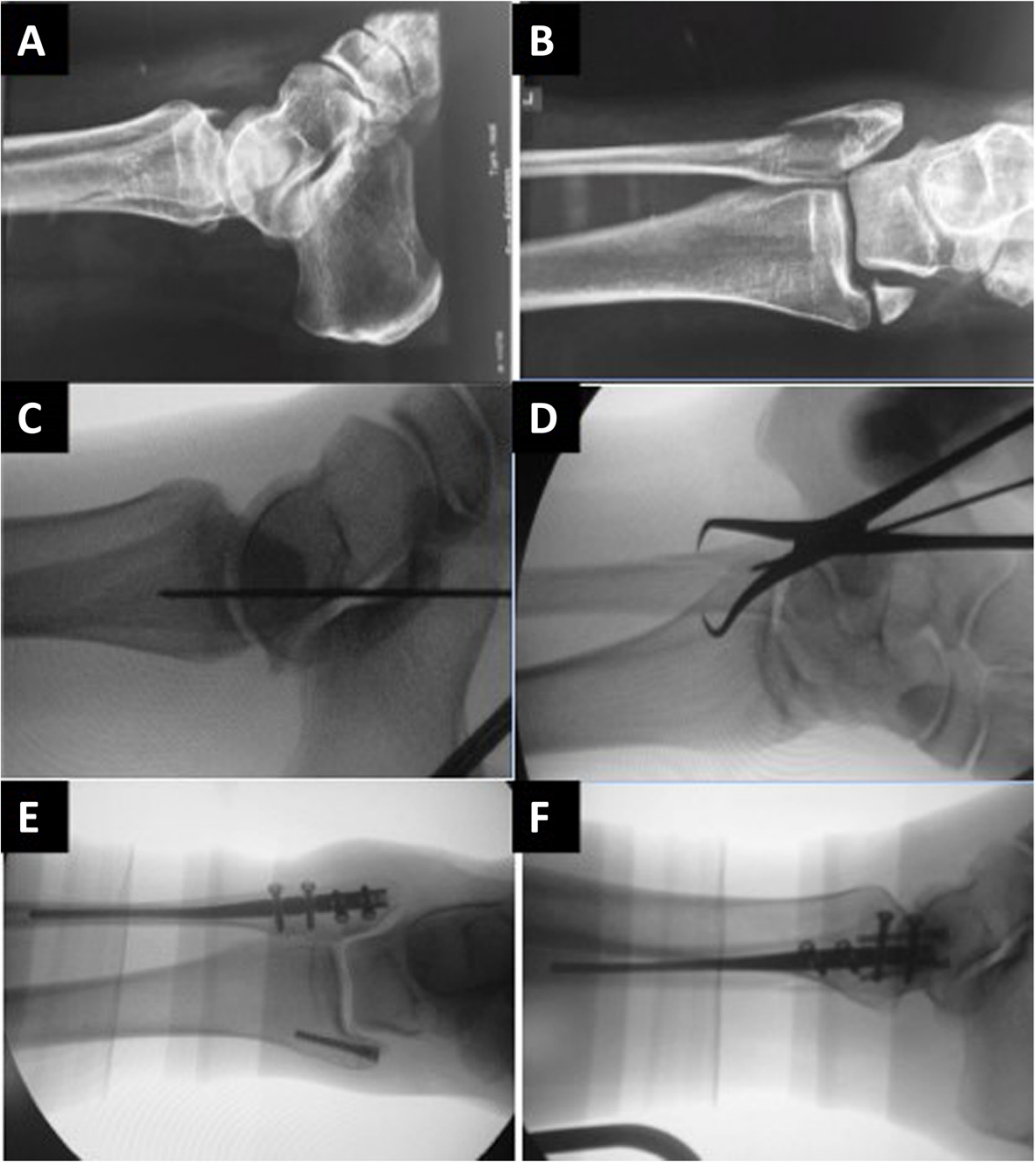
Lateral and anteroposterior radiographs of the ankle in a patient with a displaced bimalleolar ankle fracture (1A-B). Intraoperative fluoroscopic images showing the passage of the guidewire at the tip of the lateral malleolus and reduction with a percutaneous reduction forceps (1C-D). Fluoroscopic images documenting acceptable fracture reduction and appropriate implant position (1E-F)
